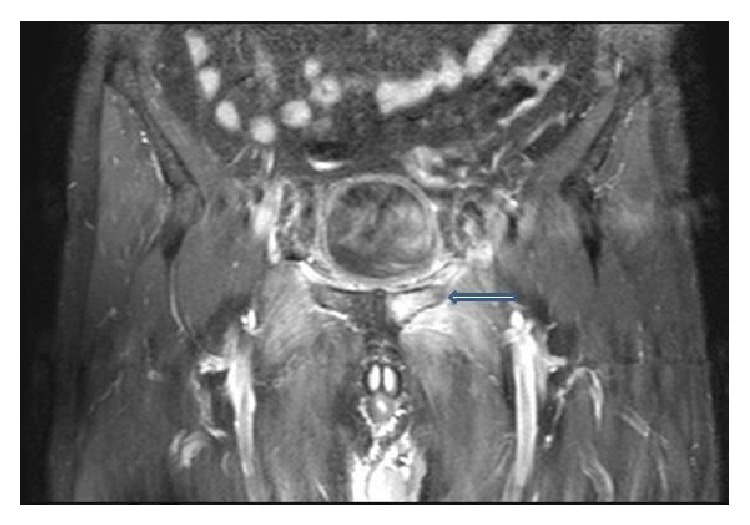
Pelvic MRI demonstrated inflammatory change in left pubic bone (arrow) and adductor muscle.
